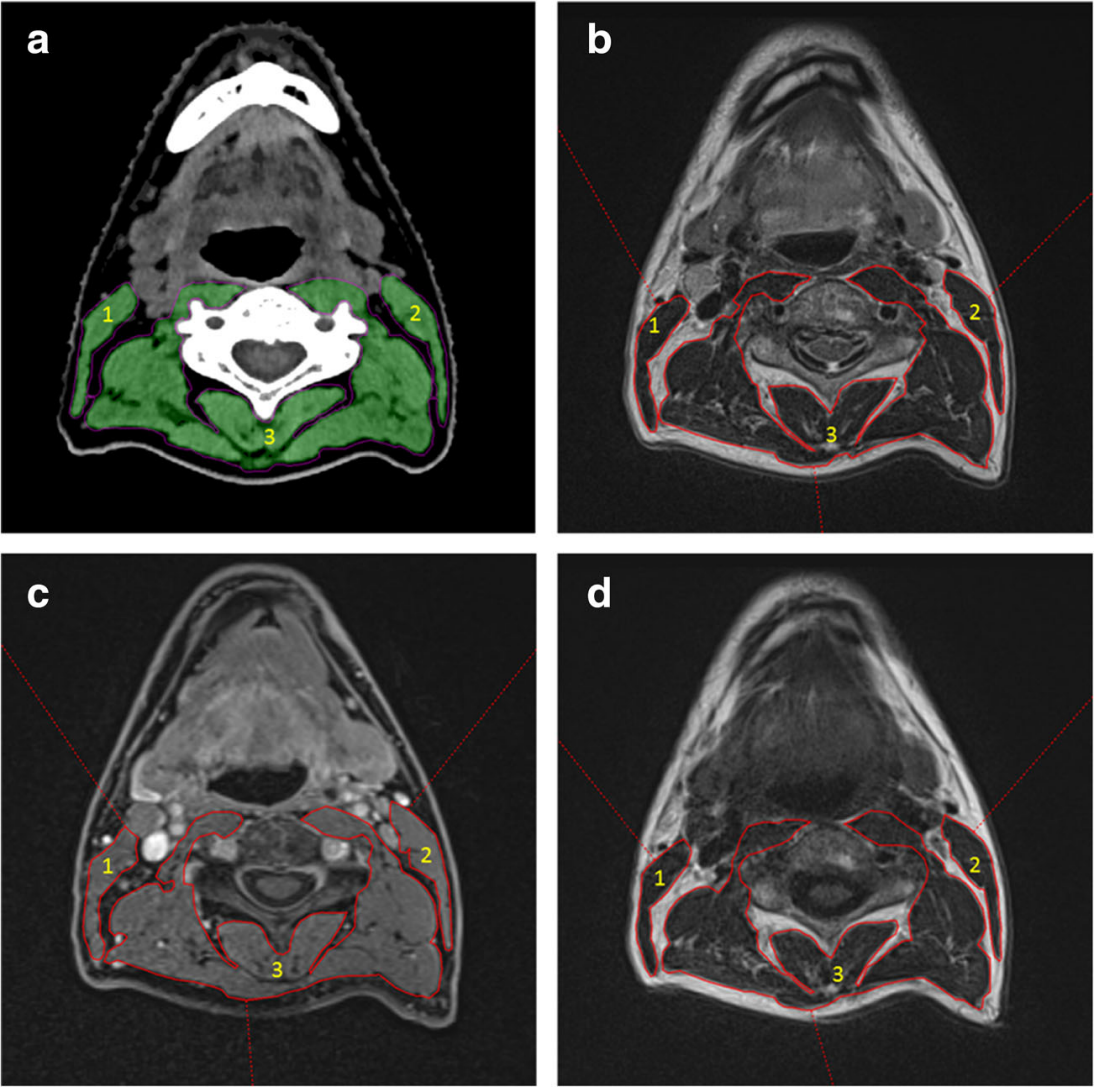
Skeletal muscle measurements at the level of C3 on axial neck CT and MRI images. (1) CSA of the right sternocleidomastoids muscle, (2) CSA of the left sternocleidomastoids muscle, and (3) CSA of the paravertebral muscles. a Acquired using CT. The green area represents the delineated muscle area which corresponds with the preset Hounsfield units range of − 29 until 150. Other densities are automatically excluded from the measured area. b–d Slices acquired using 1.5-T MRI. The before-mentioned muscles are delineated red with (b) T2 without fat suppression, (c) T1 with contrast and fat suppression, and (d) being T1 sequence without contrast and without fat suppression. *C3* third cervical vertebra, *CT* computed tomography, *MRI* magnetic resonance imaging, *CSA* cross-sectional area
